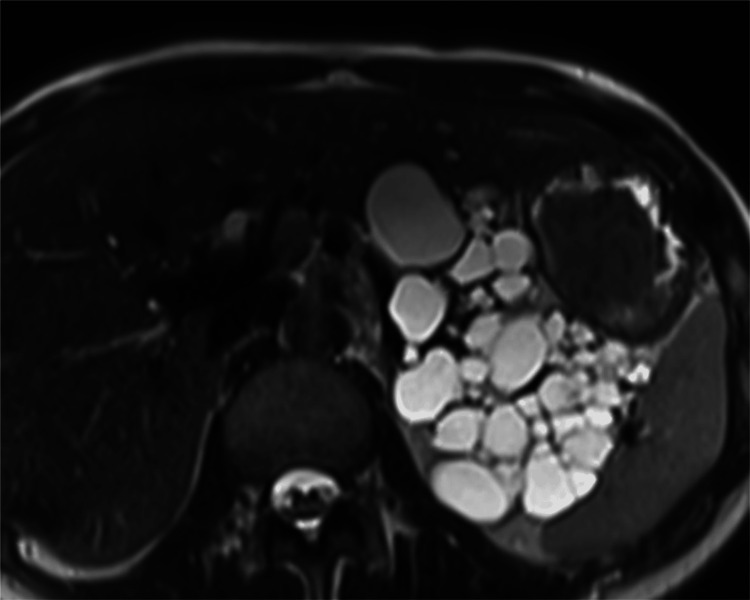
An axial heavily T2-weighted magnetic resonance image of a 25-year-old woman without diabetes mellitus or a high HbA1c level This patient is different from the patient in Figure 1. The percentage of pancreatic cysts is 90.8%.
